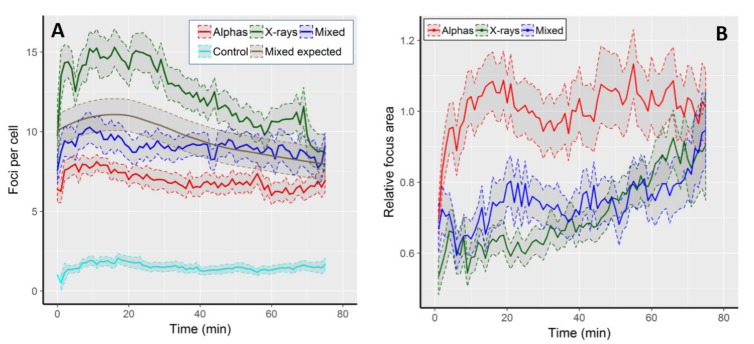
(A) Kinetics of 53BP1 focus frequency in control cells and following exposure to alpha particles, X-rays and mixed beams. The expected frequencies following mixed beam exposure were calculated as half of the sum of corresponding values from alpha and X-ray exposed cells, and are shown as a smooth grey curve. (B) Average, relative focus areas in cells exposed to alpha particles, X-rays, and mixed beams. Focus area is expressed as fraction of the respective control value. Shaded areas represent standard error of the mean.
